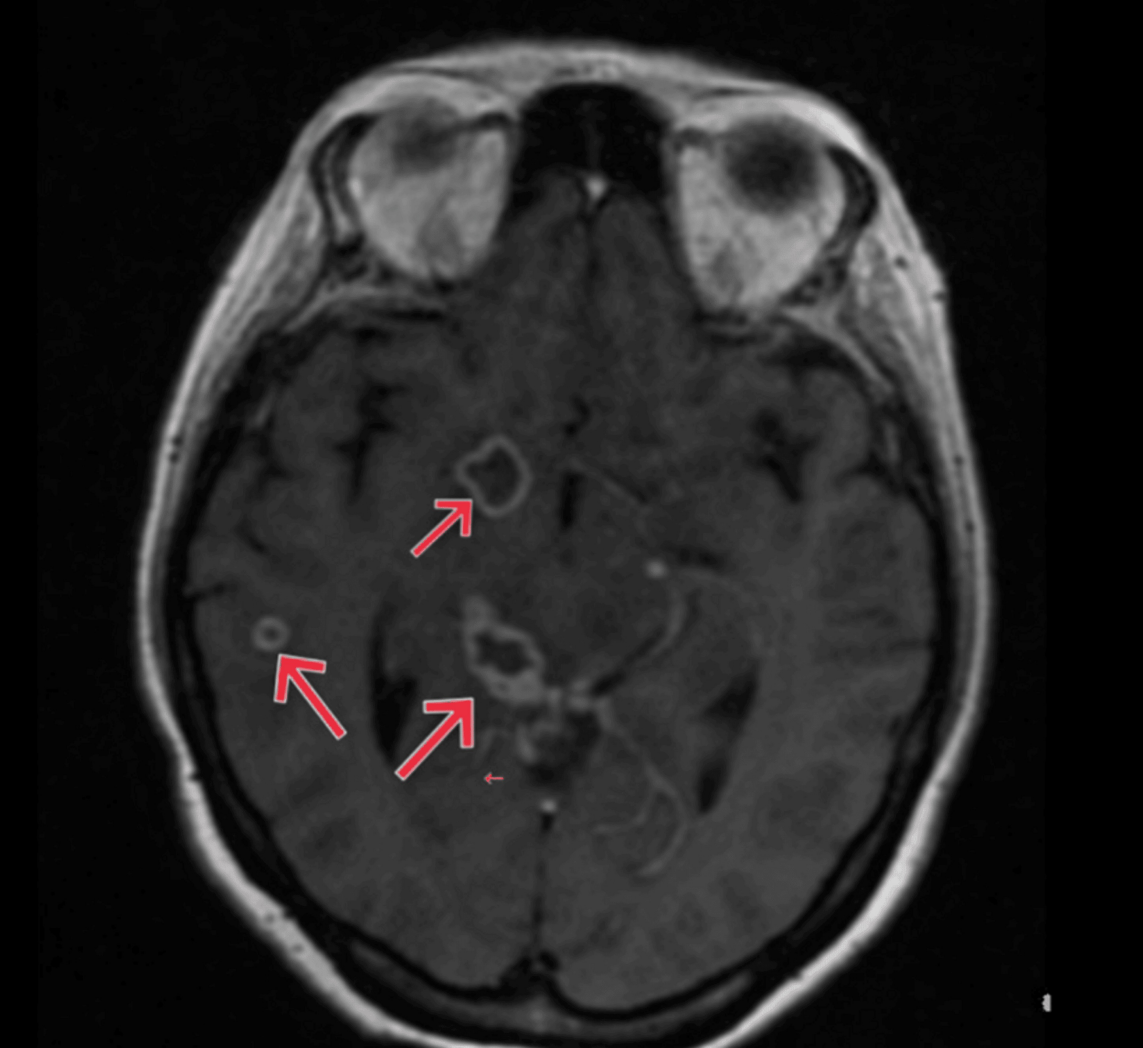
Axial post-contrast T1-weighted MRI showing multiple ring-enhancing lesions in the right cerebral hemisphere with surrounding vasogenic edema.
